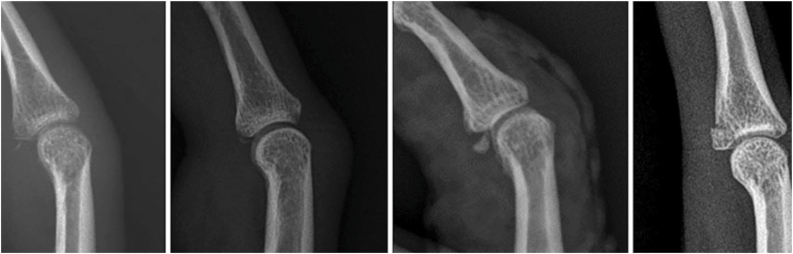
Lateral radiograph showing a sliver-shaped volar plate avulsion fragment at the base of the middle phalanx. Volar plate avulsion fractures may appear as a dot, sliver, triangle, or rhomboid fragment on lateral views.
